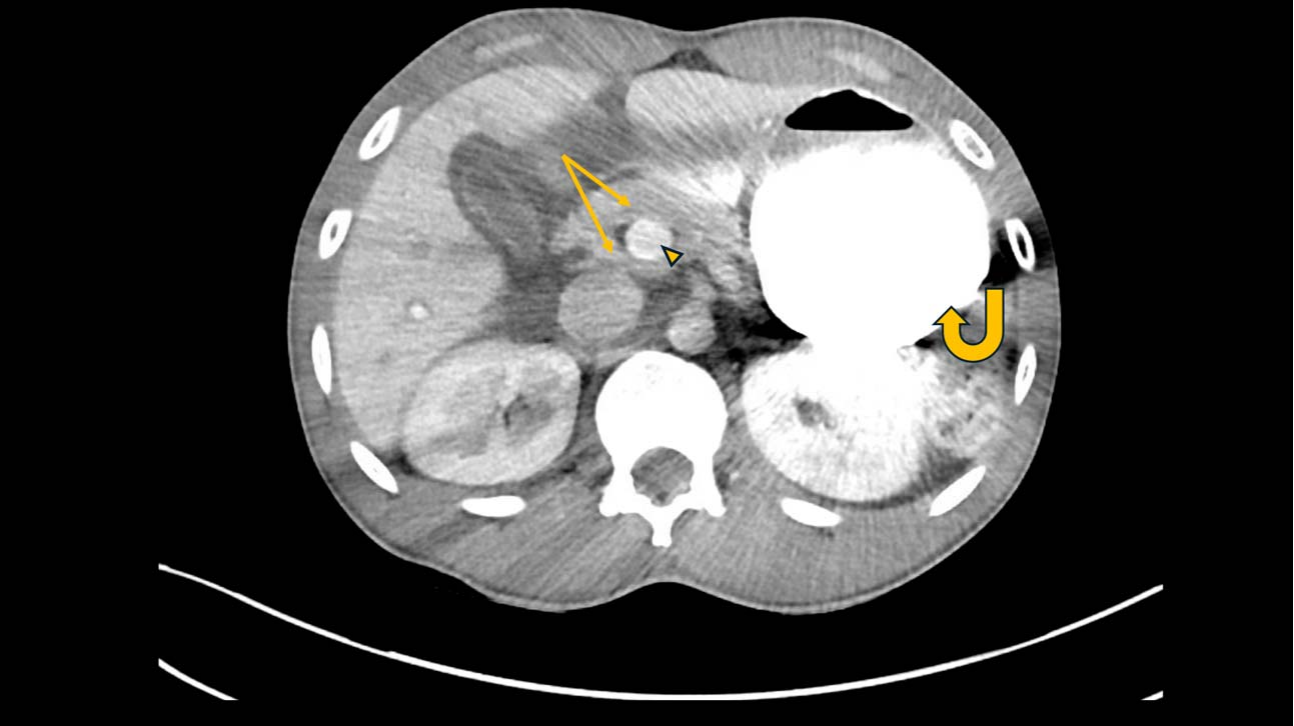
Contrast-enhanced axial abdominal computed tomography scan highly suggestive of annular pancreas configuration (arrows), encircling the second portion of the duodenum (arrowhead), and a distended stomach (curved arrow).
